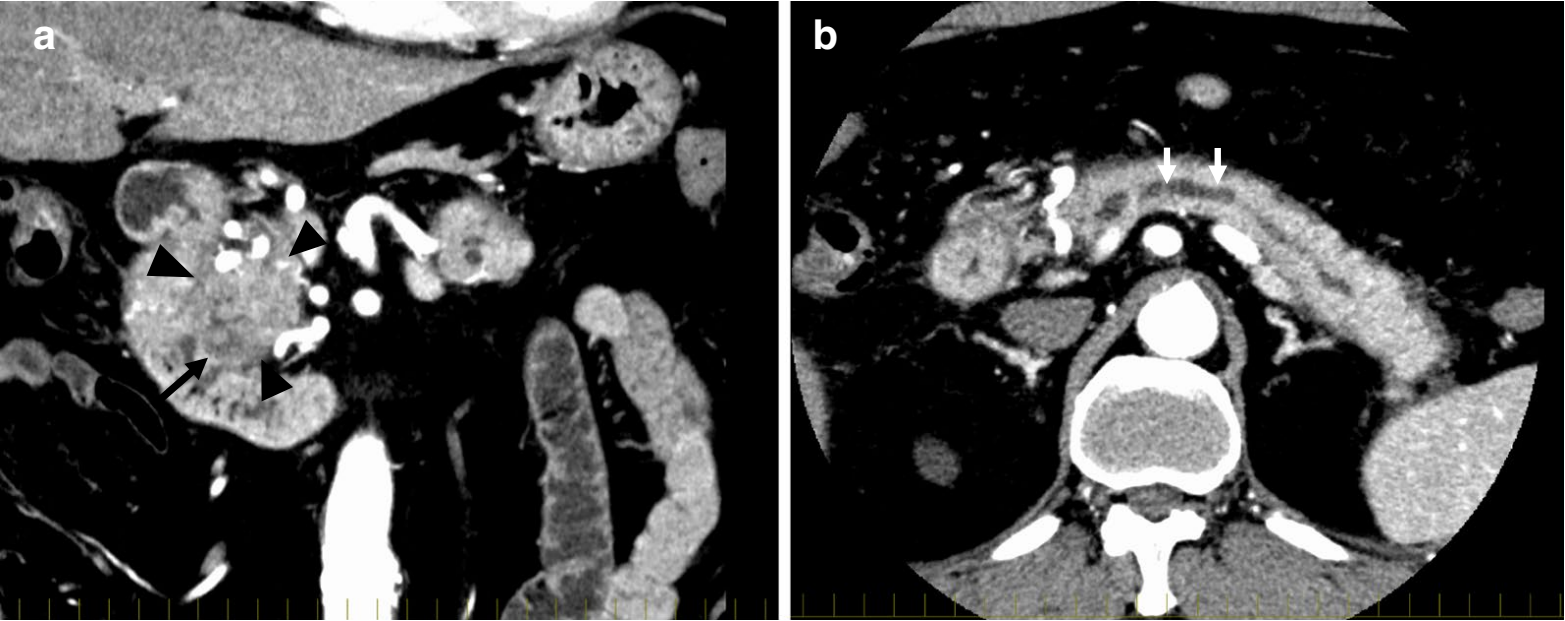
Contrast-enhanced CT. a A 40-mm mass with an irregular margin was detected in the pancreatic head (arrowhead), and a low-density area with 20 mm in diameter was observed on the duodenal side of the mass (arrow). b An expanding main pancreatic duct was detected with a 9-mm diameter at the distal side of the tumor (arrow)
